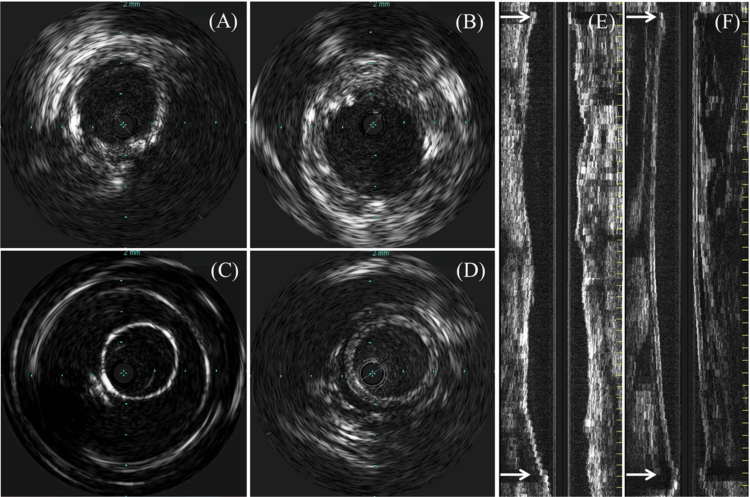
IVUS evaluation of implanted grafts. Intravascular ultrasound (IVUS) images in cross-sectional and longitudinal views on days 0 (A, C) and 14 (B, D–F). TESG (A, B, and E) preserved luminal architecture, whereas ePTFE grafts (C, D, and F) showed mild luminal thickening and stenosis at the distal anastomosis. Arrows indicate anastomotic sites.
